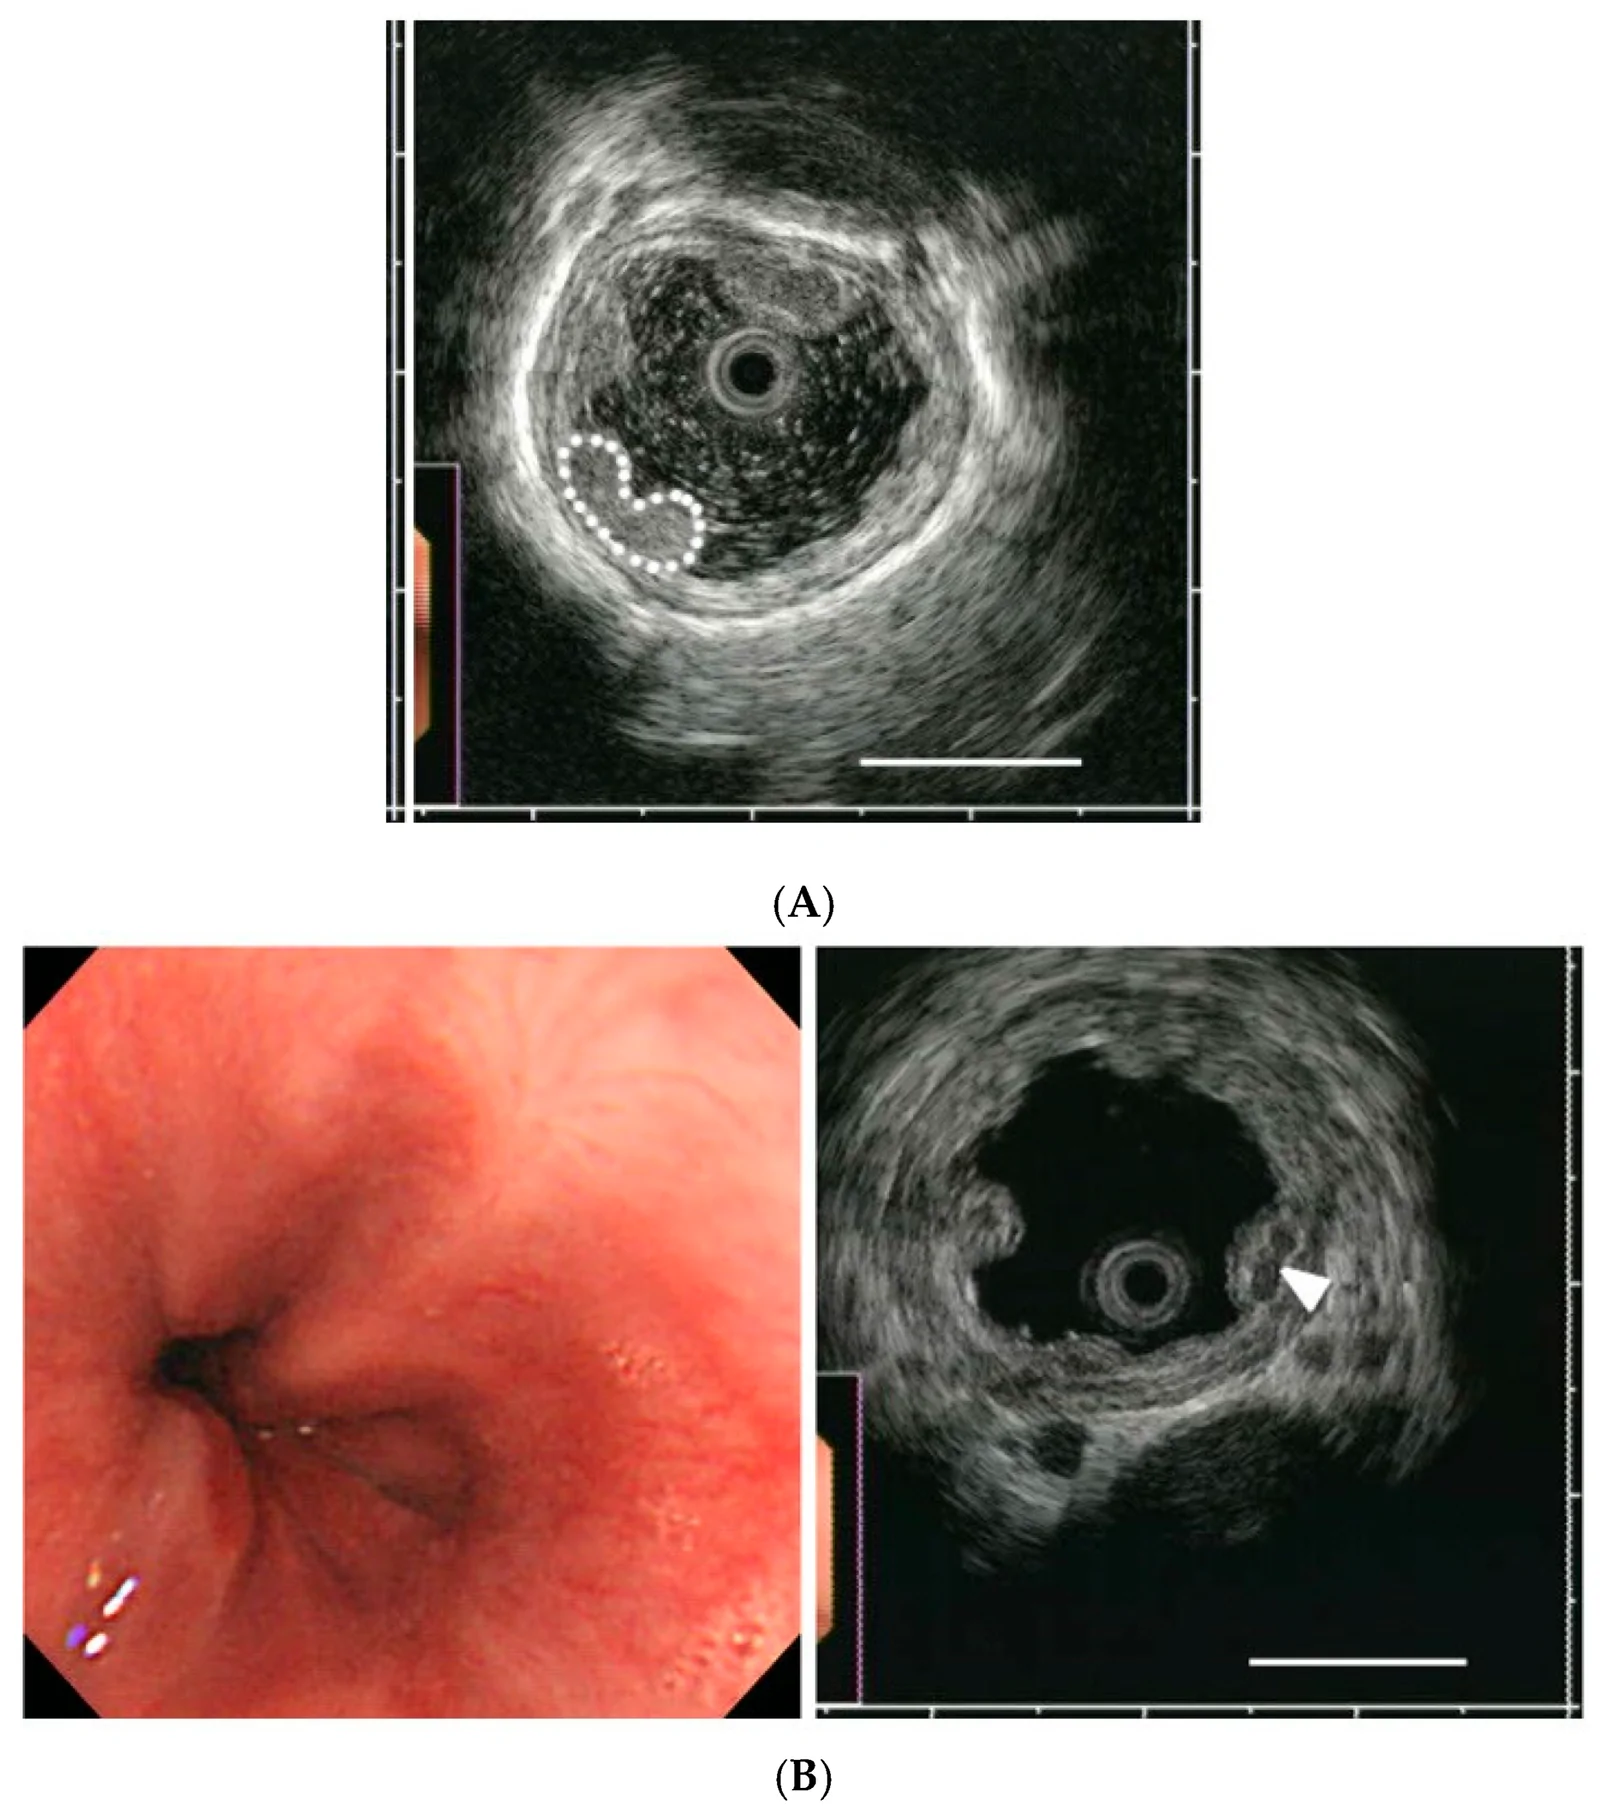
(A) Endoscopic ultrasonography (EUS) image using high-frequency intraluminal ultrasound (HFIUS) of post-endoscopic variceal ligation (EVL) esophageal varices. The dotted line indicates the largest variceal cross-sectional area (CSA). Scale bar = 10 mm. (B) The varix was classified as grade 0 on esophagogastroduodenoscopy (EGD), whereas EUS using HFIUS demonstrated a residual variceal CSA of 6.23 mm^2^. The arrow indicates the residual varix detected by HFIUS.
